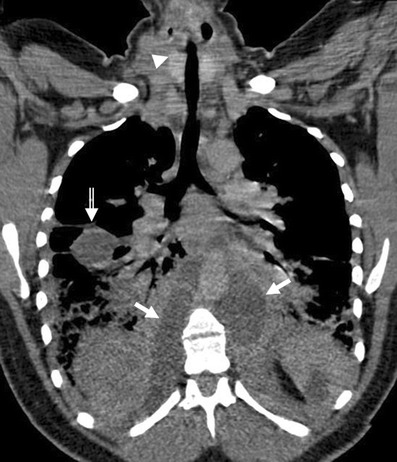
Oblique-coronal multiplanar reconstruction of contrast-enhanced CT scan of a 33-year-old woman with a descending necrotising mediastinitis. Hypodense para-aortic areas correspond with fluid-collections (*arrows*) that extend to the retroperitoneum. Pleural effusion in the fissure is also be seen (*open arrow*). Note the visceral space involvement (*arrowhead*)
